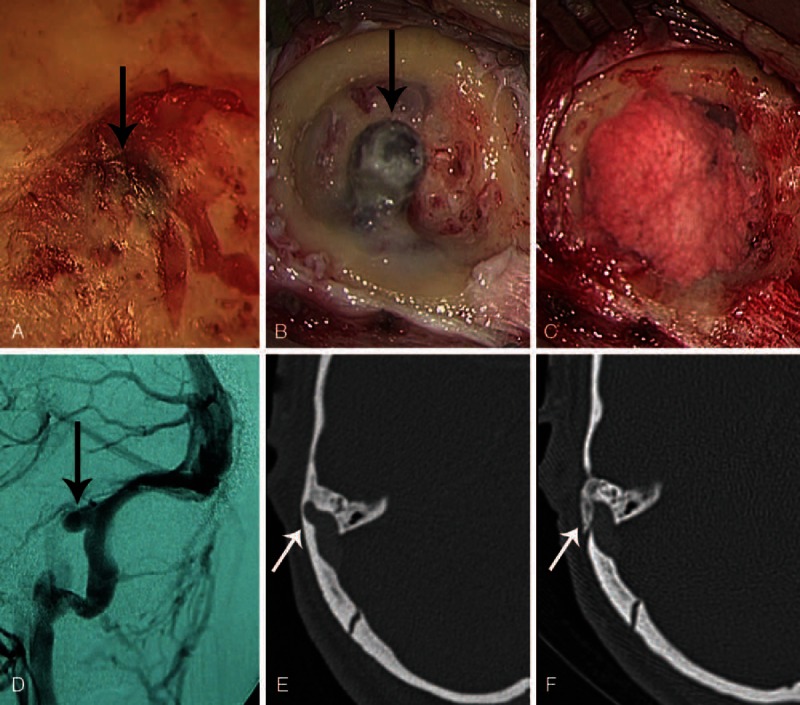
Intraoperative photographs (A–C) and preoperative (D and E) and postoperative (F) imaging findings for a patient (Patient 4) experiencing the complete resolution of PT following surgery. A, The diverticulum is shown through the thin, overlying mastoid cortex (arrow). B, The diverticulum is isolated following skeletonisation (arrow). C, The sigmoid sinus wall is reconstructed with fascia and autologous bone pate. D, Preoperative DSA image of the diverticulum (arrow). E, Preoperative CTA shows the diverticulum protruding into the adjacent mastoid region (arrow). F, Postoperative CTA, at the same level as in (E), demonstrates the elimination of the diverticulum, with a normal diameter of the sigmoid sinus (arrow).
